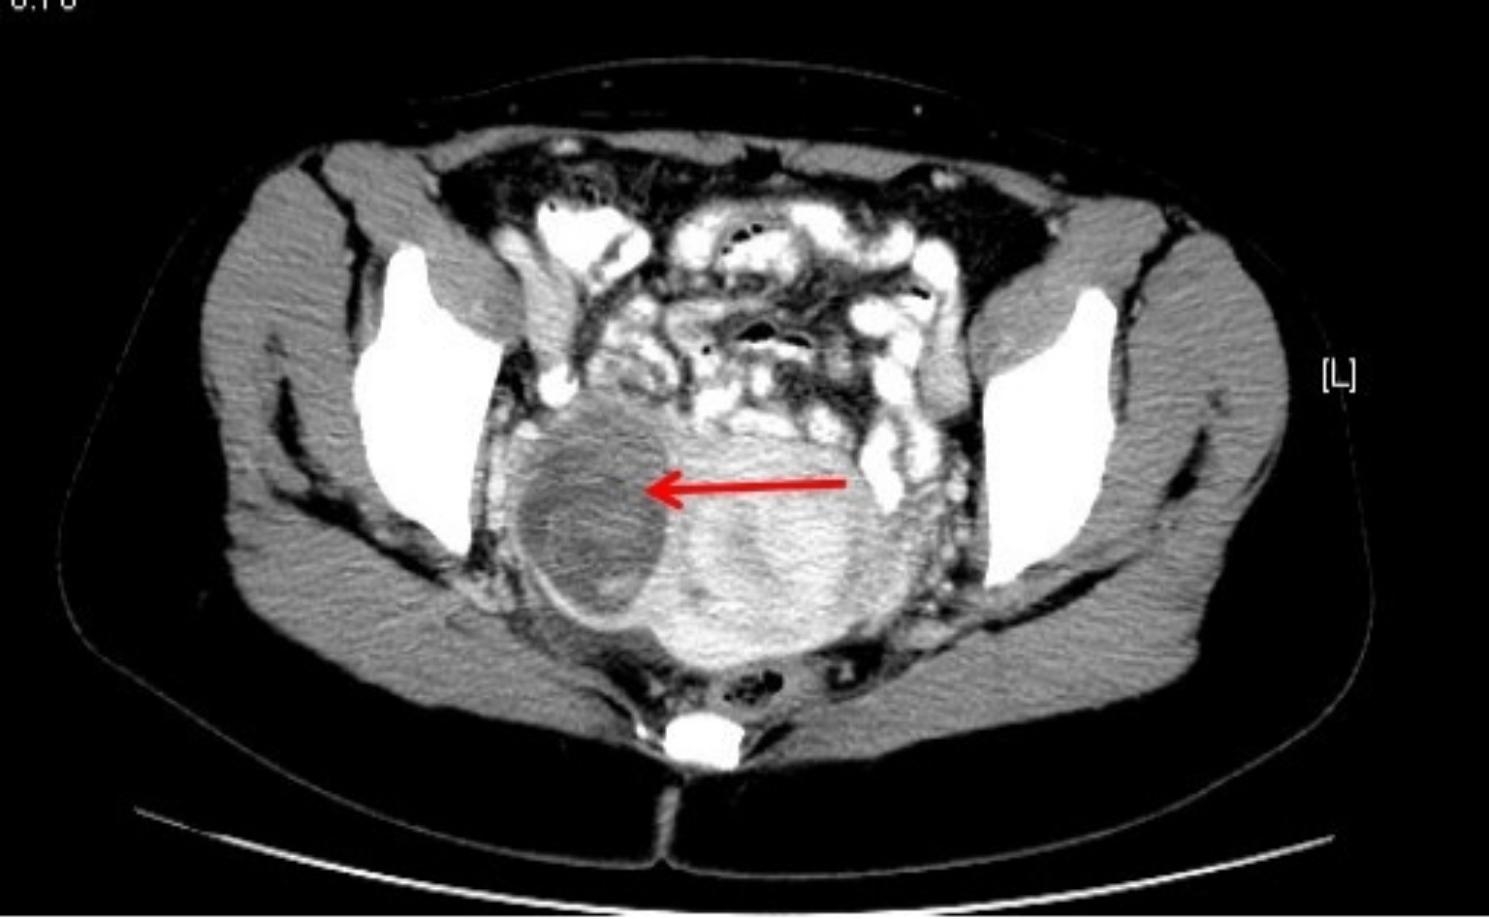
CT showed a round low-density soft tissue mass (red arrow) with a size of approximately 46*40 mm beside the right lateral wall of the myometrium
